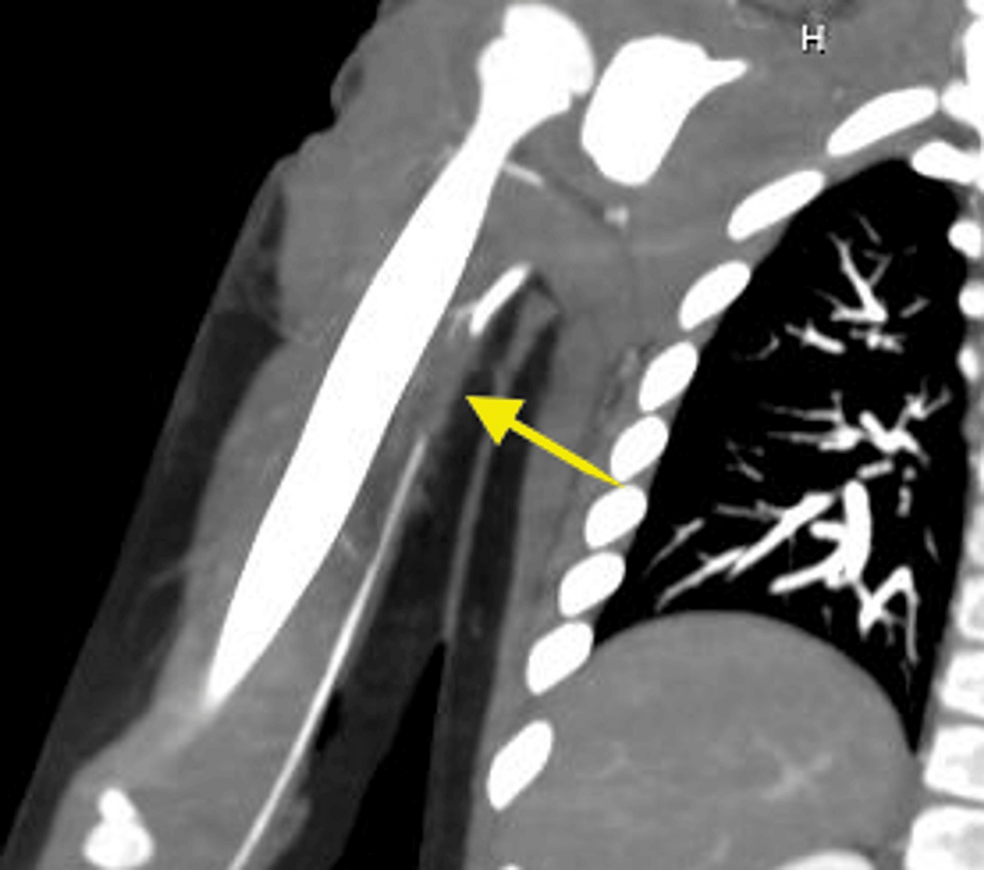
Proximal right brachial artery occlusion without evidence extravasation shown on CTA. The occlusion is 2.5cm in length with reconstitution of the distal brachial artery.
